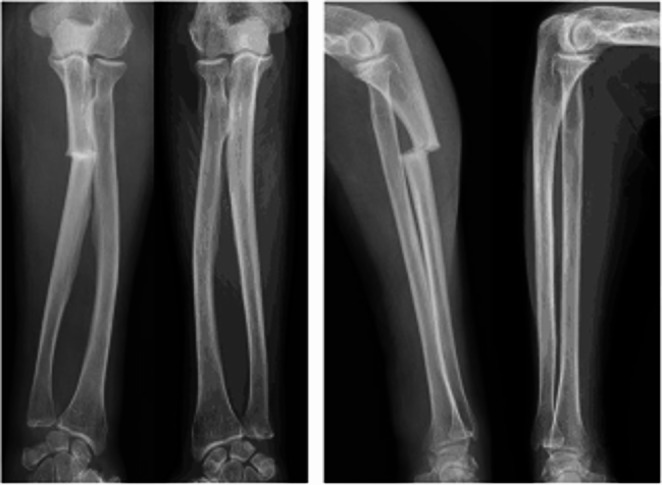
Preoperative radiography of bilateral forearm demonstrating left ulnar shaft fracture. *(Courtesy of BMC Musculoskelet Disord).*
